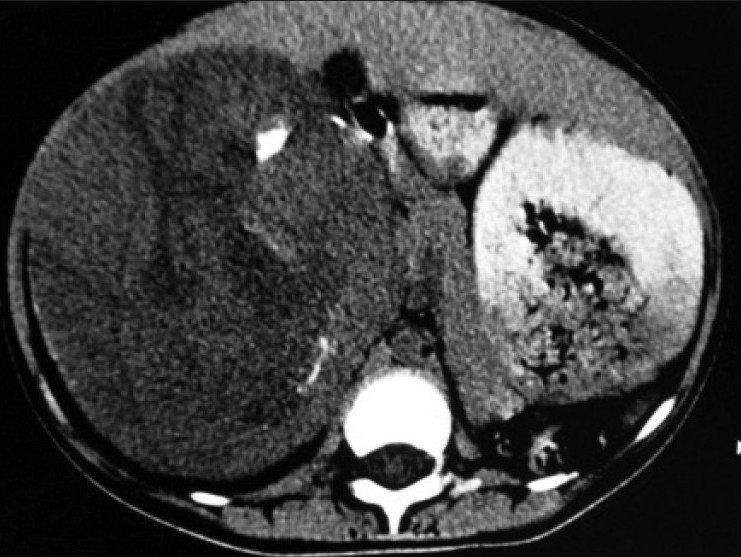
Non-contrast enhanced CT scan revealed large heterogenous mass with few chunks of calcifications
